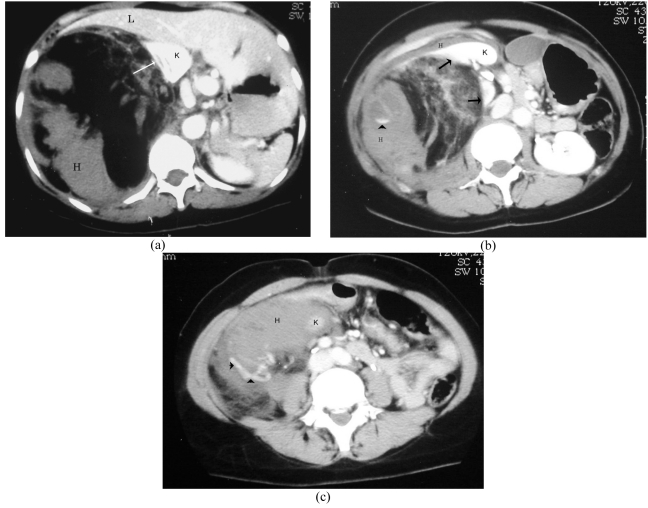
Enhanced-CT images at (A) upper pole (B) middle pole, and (C) lower pole of the right kidney (K) show a 13x15x20 cm fat-containing mass arising from the lateral aspect of the kidney with sharp defect (arrows) in renal parenchyma, enlarged vessels (arrowheads), intratumoural and perinephric hemorrhage (H), and compression of the liver (L).
